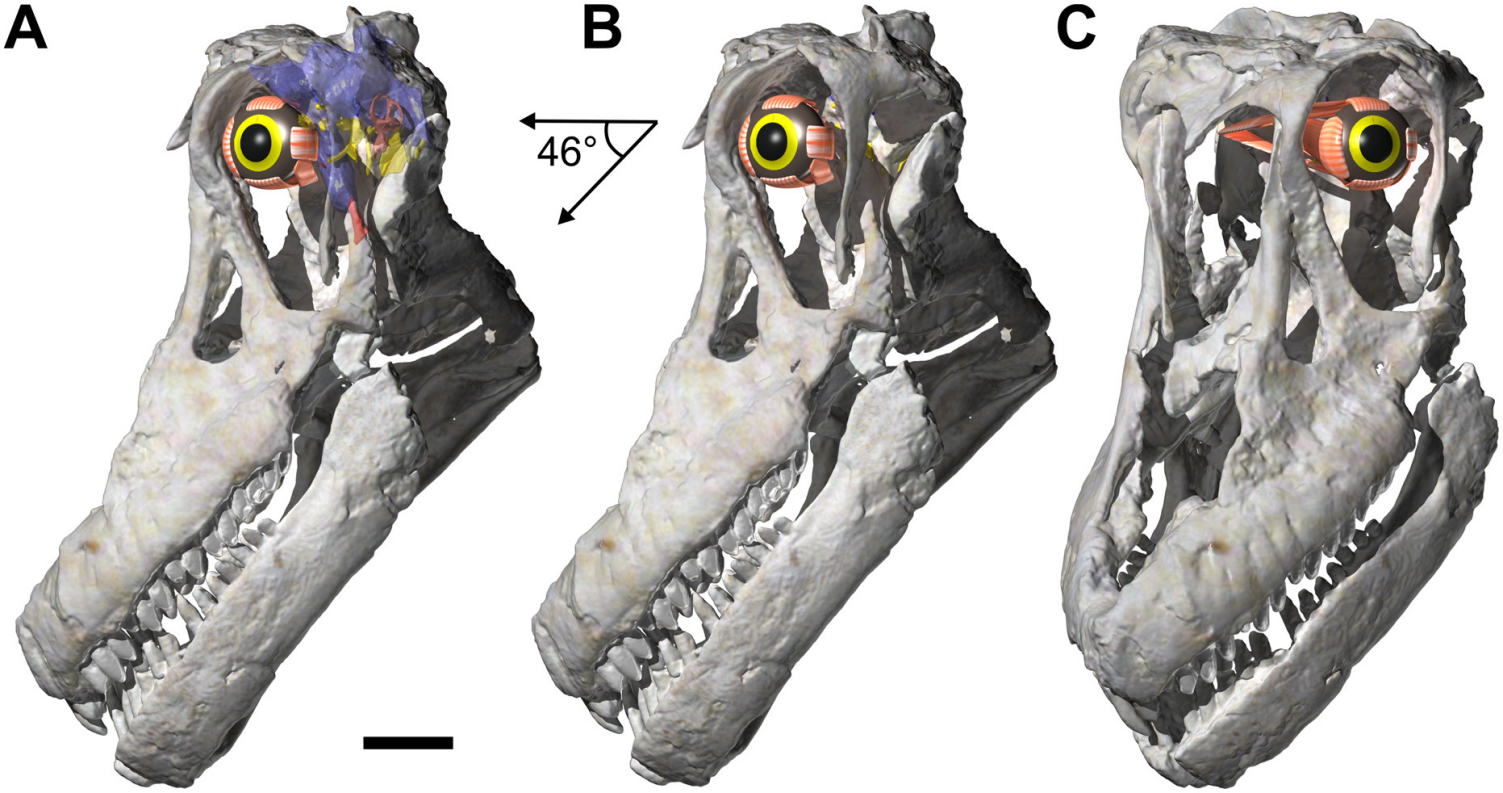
Computed tomography-based digital visualizations of the skull of *Sarmientosaurus musacchioi* gen. et sp. nov. (MDT-PV 2) in the ‘alert’ posture. (A) Skull rendered semitransparent in left lateral view, revealing the cranial endocast. (B) Skull rendered solid in left lateral view. (C) Skull rendered solid in left rostrolateral view. With the lateral semicircular canals oriented roughly with Earth horizontal, the skull is oriented with the tooth row 46° below horizontal. The eyeball is restored to provide a sense of the impact of the the strongly downturned ‘alert’ head posture on the visual field. Although the bony scleral ring was not preserved in *Sarmientosaurus*, its dimensions were based on scaling the complete ring of the titanosaur *Nemegtosaurus mongoliensis* (ZPAL MgD-I/9) and using known osteological correlates preserved n MDT-PV 2. Scale bar = 5 cm.
